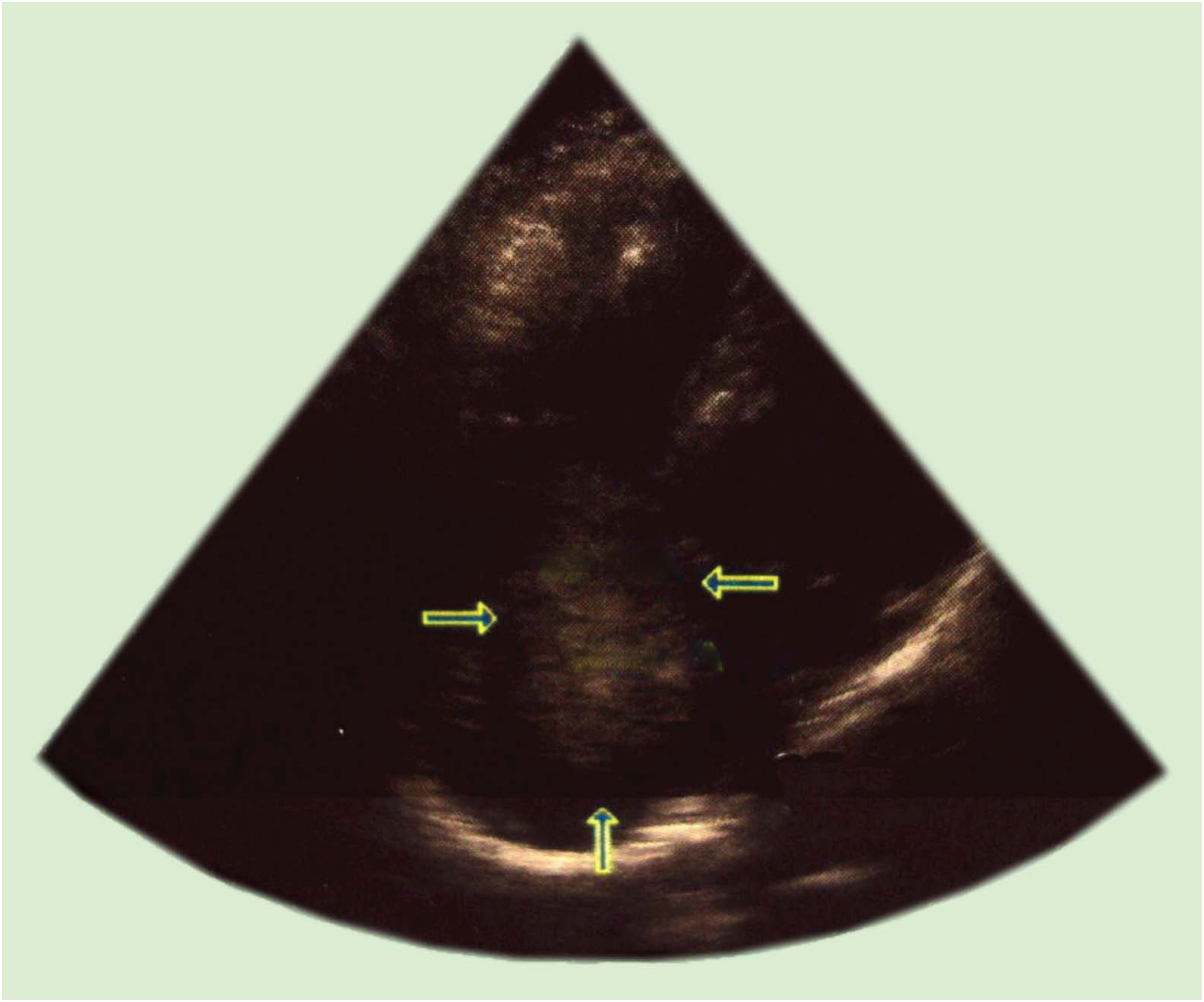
A transthoracic echocardiogram shows a mass in the right atrium before an operation.
